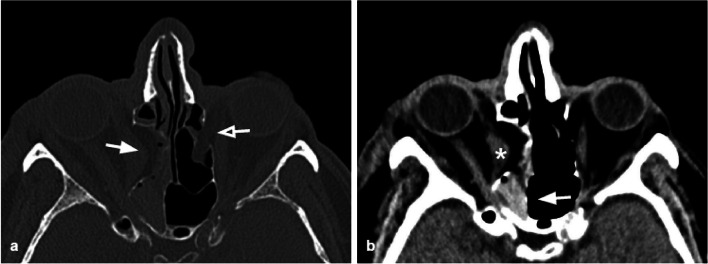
Axial CT acquisition with bone (a) and soft tissues (b) algorithms in a patient with a current fracture of the right orbital medial wall (white arrow) and a previous fracture of the contralateral orbital medial wall (empty arrow). a Extensive discontinuation of the right lamina papyracea (white arrow). b The medial rectus muscle is swollen, displaced medially, and partially entrapped into the fracture (asterisk); presence of hemosinus, visible as a right posterior ethmoidal cell occupied by hyperdense material (blood) (arrow)
